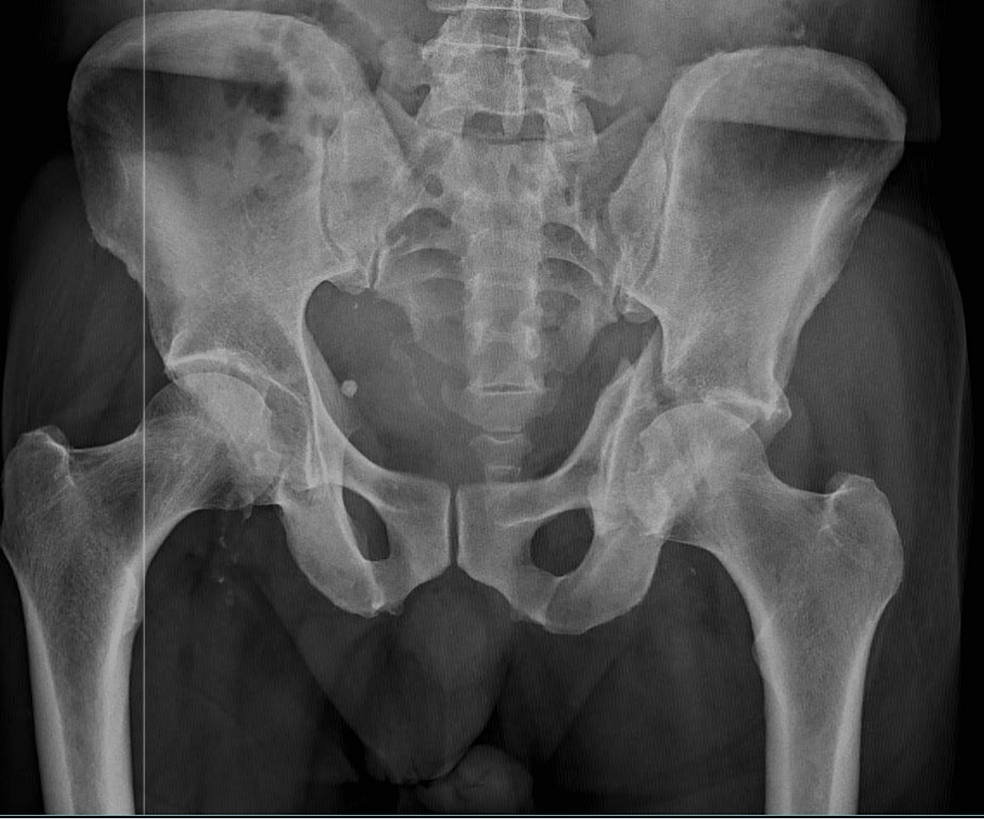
Anterior-posterior X-ray of the pelvis showing non-union of a left acetabular fracture A radiograph of the pelvis of both hips showed that the anterior column and wall had healed together, whereas the posterior column had not healed and showed medial migration and distortion of the femoral head due to an impacted fracture
